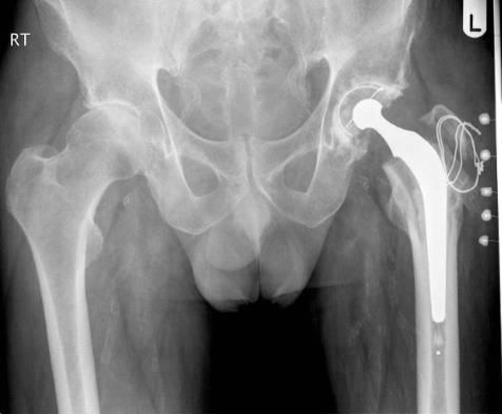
Post Charnley left total hip replacement plain X-ray of the pelvis.
